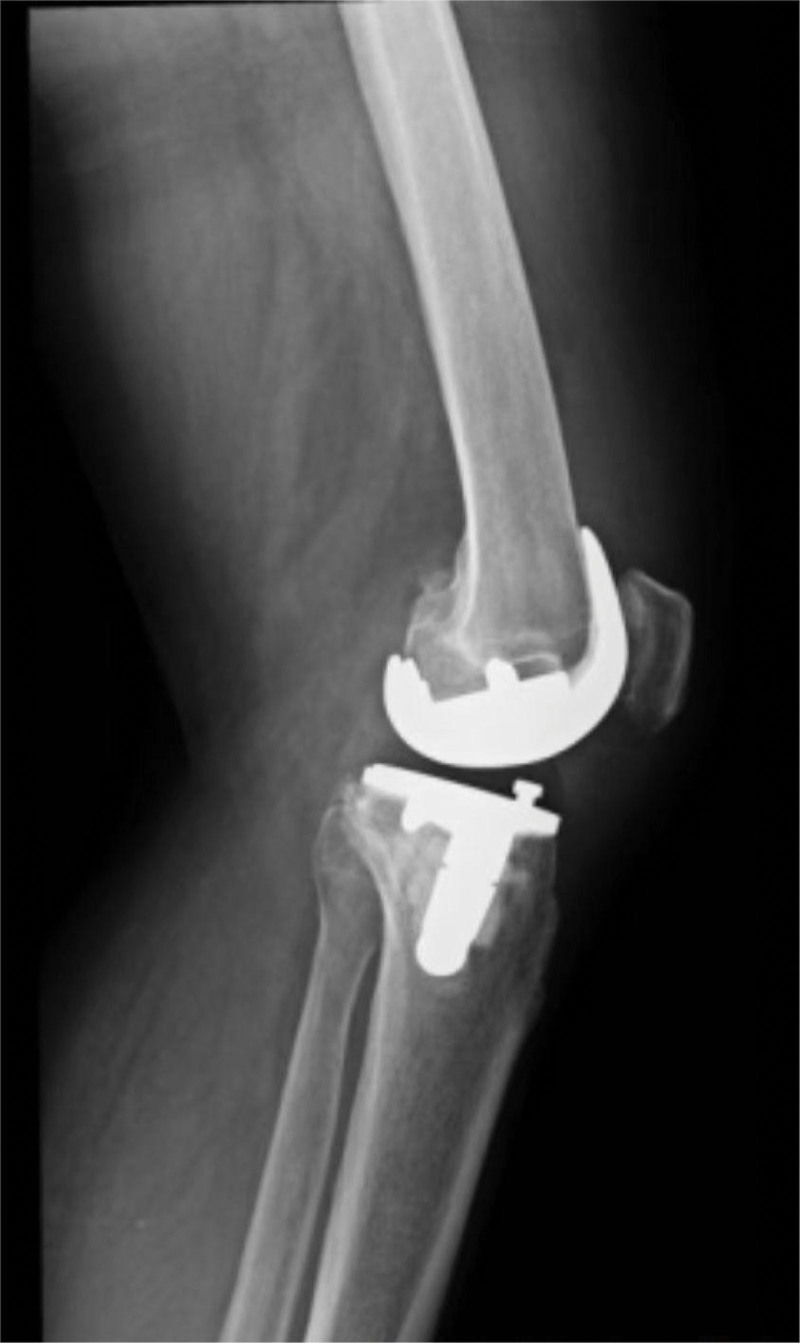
X-ray after 1 yr shows prosthesis are in good condition.
